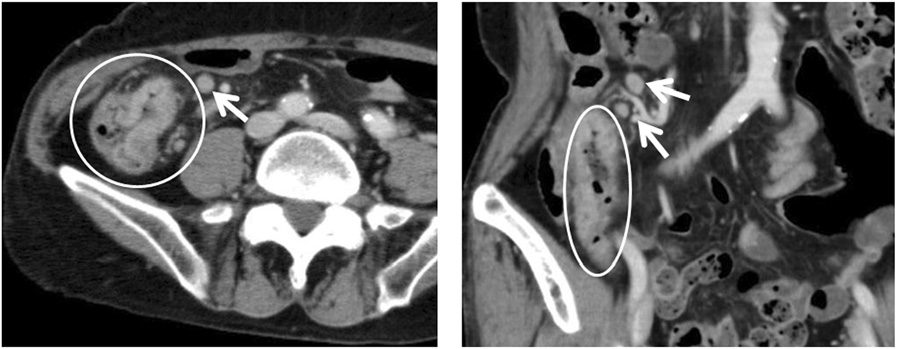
Her abdominal and pelvic enhanced CT revealed mucosal thickness of cecum (circle) and enlarged lesional lymph node (arrows). Left panel indicates transverse imaging and right panel indicates coronal imaging.
